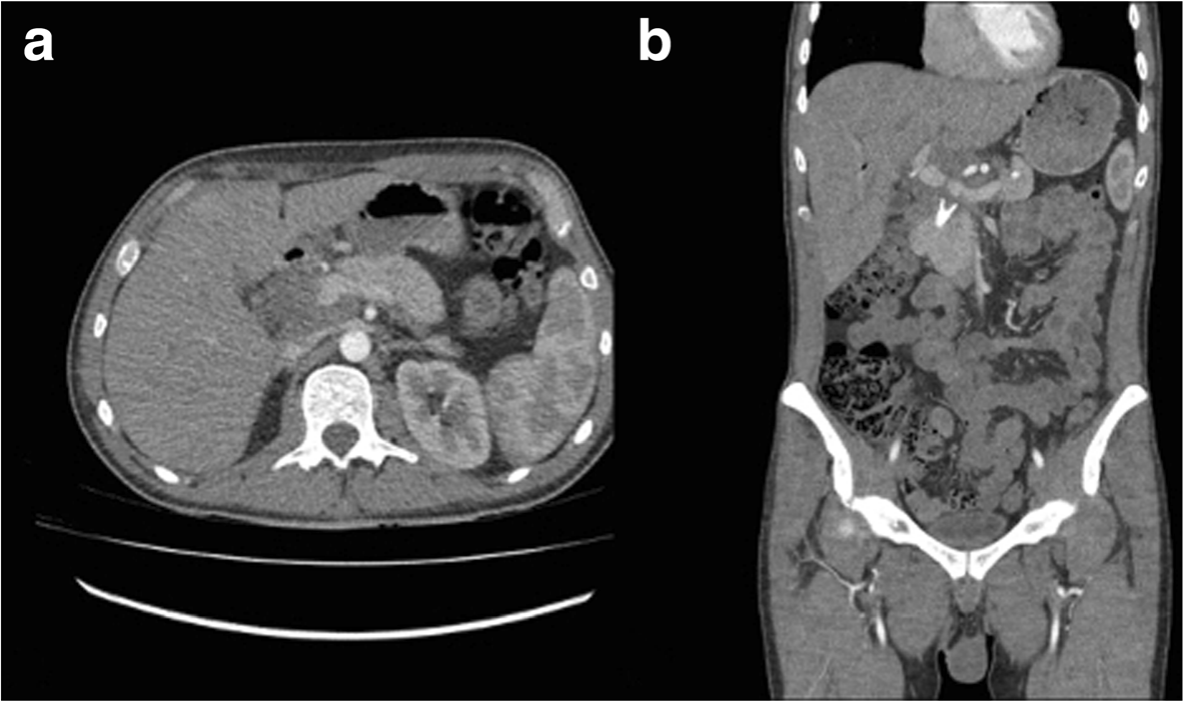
Portal venous phase CT showing a main portal vein pseudoaneurysm closely related to the upper end of the biliary stent
